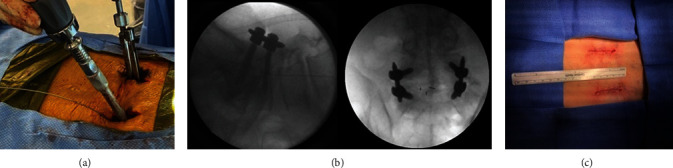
(a) Minimally invasive instrumented TLIF after bilateral pars decompression. (b) Final X-rays. (c) Wound closure.
